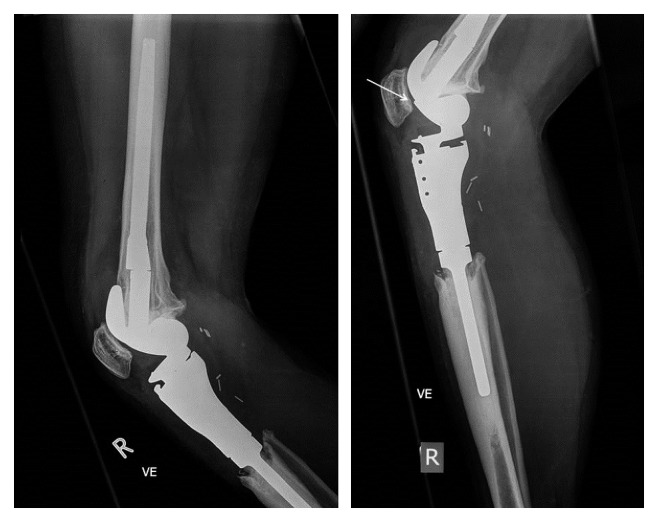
X-rays of the right knee with distal femur and proximal tibia in June 2015. Left (AP view): some bone resorption at the prosthesis-tibia interface. Intact, well-fixed prosthesis. Right: lateral X-rays demonstrated a small step (arrow) of the lateral femur condyle of the prosthesis.
